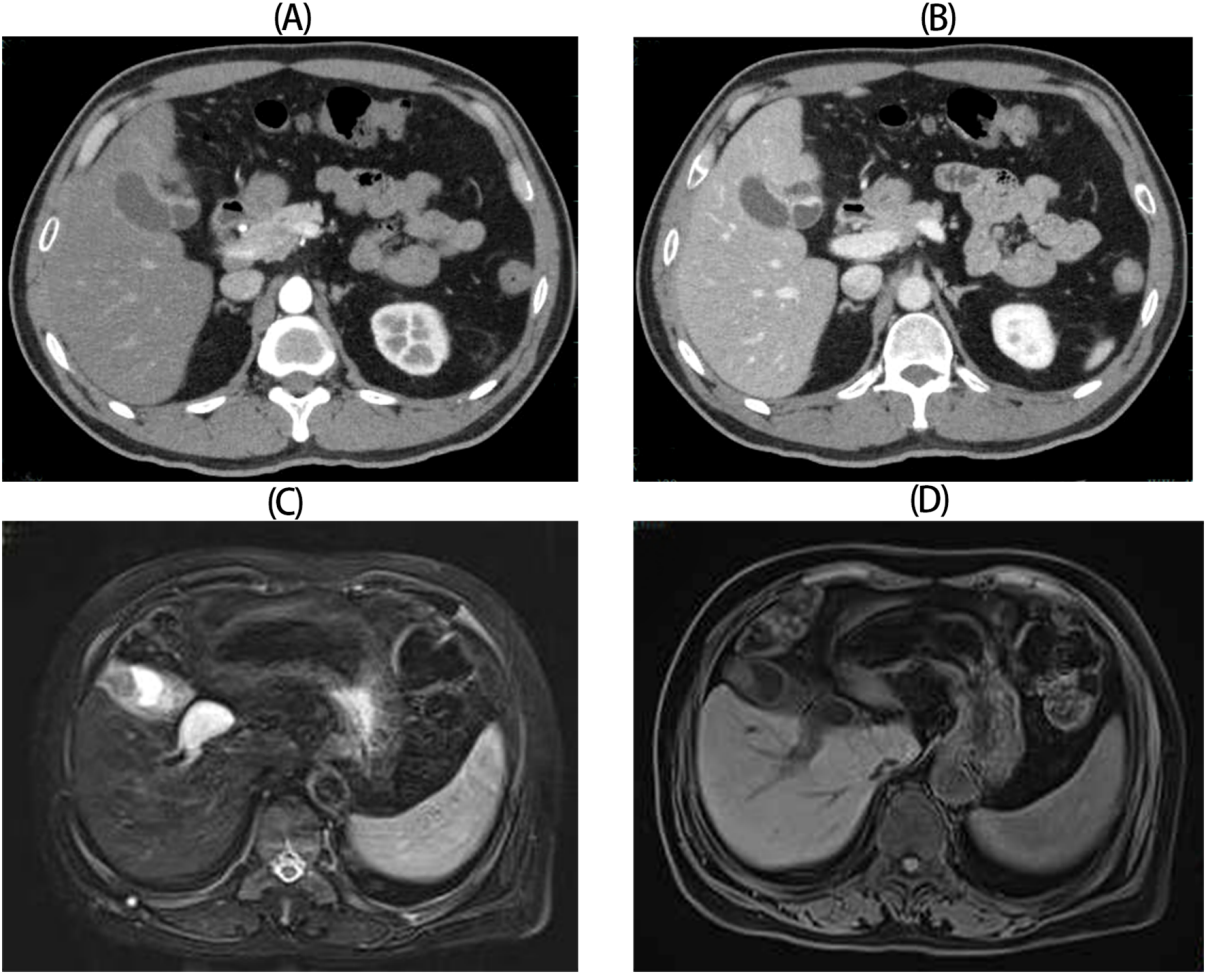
Radiologic images for GB-NENs. (A, B) Case 6: Irregular gallbladder wall thickening with arterial and venous phase enhancement (CT). (C, D) Case 5: An irregular, patchy soft-tissue signal at the gallbladder fundus (MRCP).
